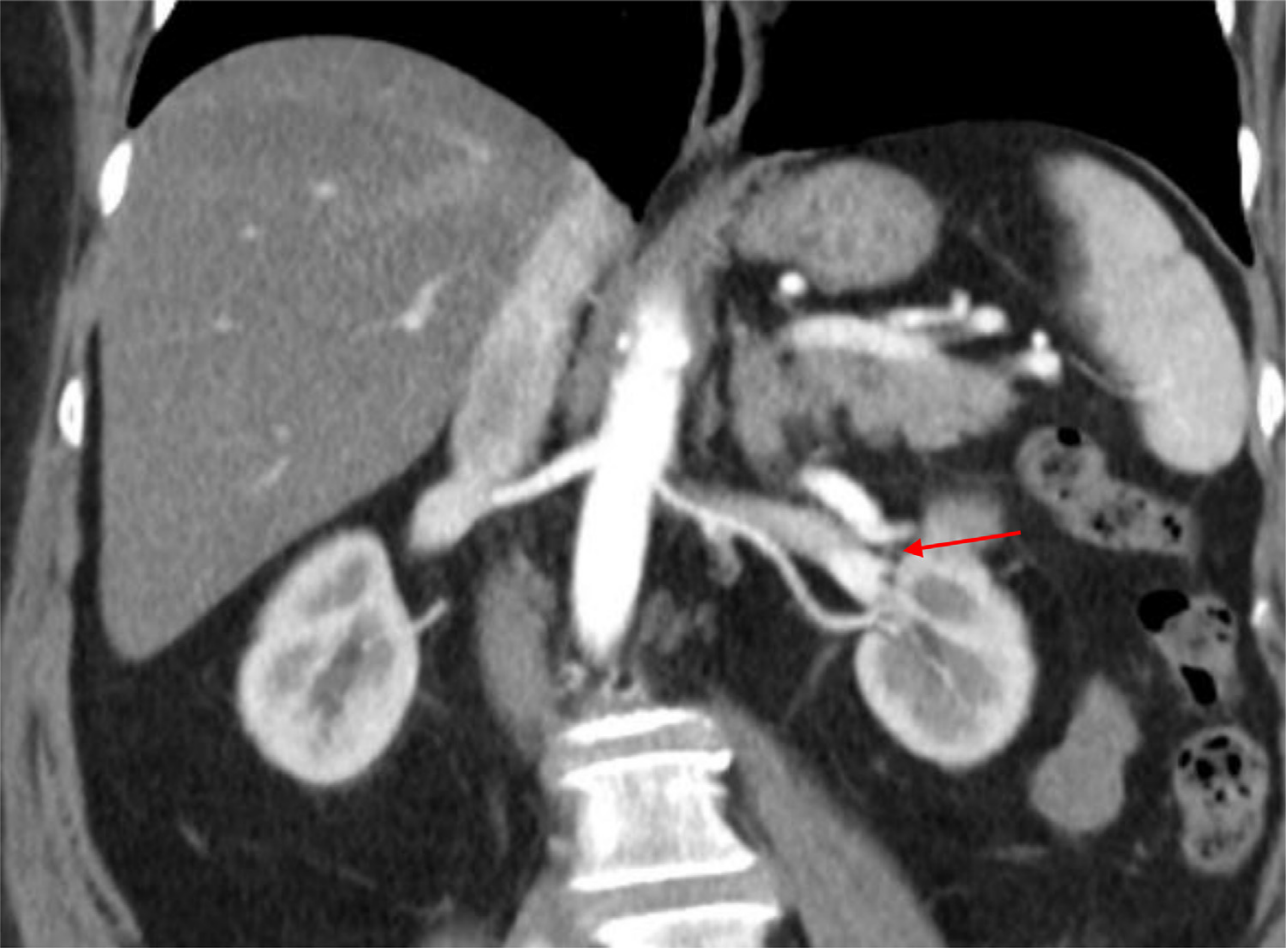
CT arterial phase angiogram shows left AVF (arrow)
